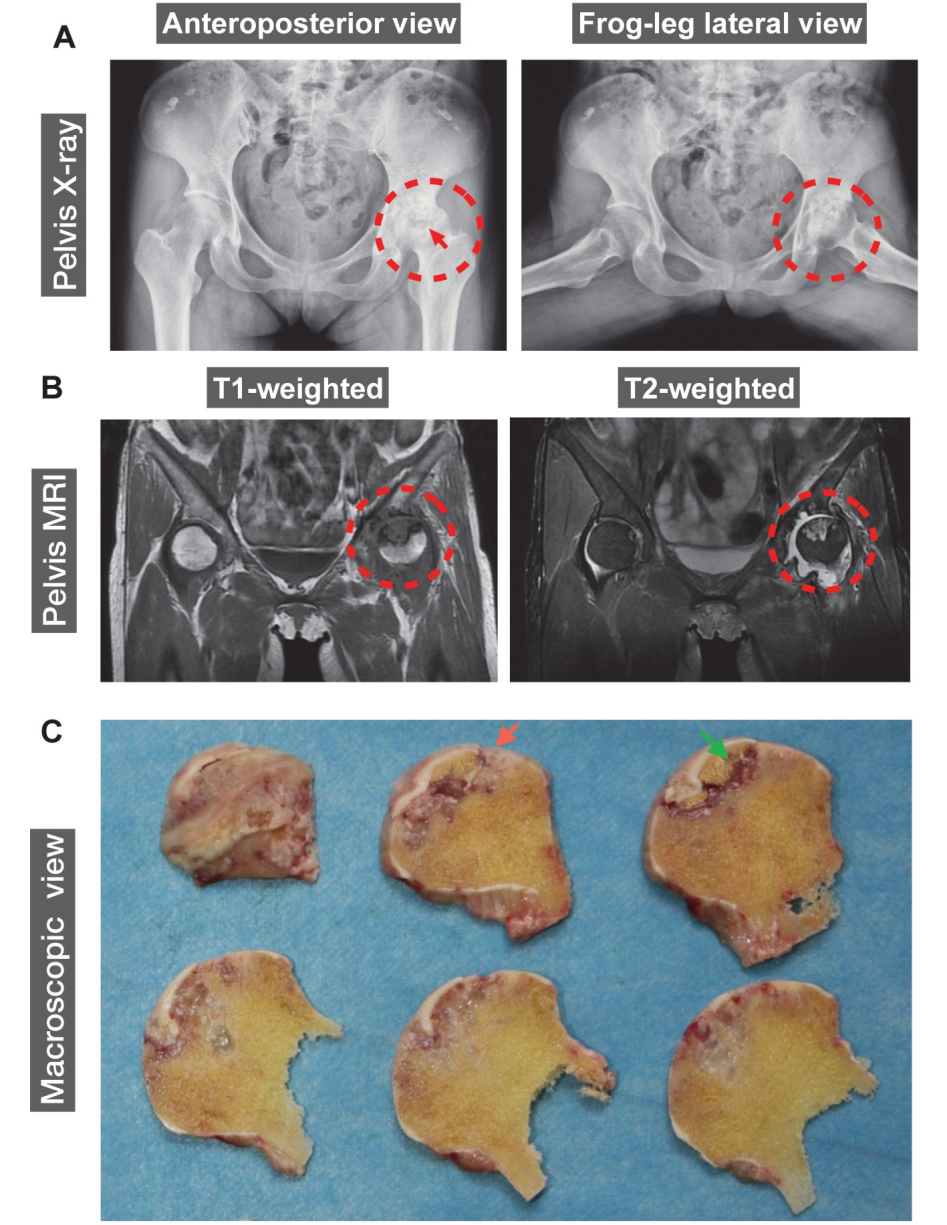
A representative 42-year-old female case of steroid-induced osteonecrosis of femoral head (ONFH, ARCO stage IV). (A) Radiographs delineating the crescent sign (red arrow), flattening of the articular surface of femoral head, and osteoarthritic acetabular changes (red dashed circles). (B) Coronal T1- and T2-weighted magnetic resonance images (MRI) highlighting a medium-sized necrotic lesion of the left femoral head (red dashed circles). (C) Gross appearance of sequentially sliced sections of the femoral head obtained at surgery, showing the deformation of the femoral head (red arrow) and subchondral necrotic bone (green arrow). ARCO, Association Research Circulation Osseous.
